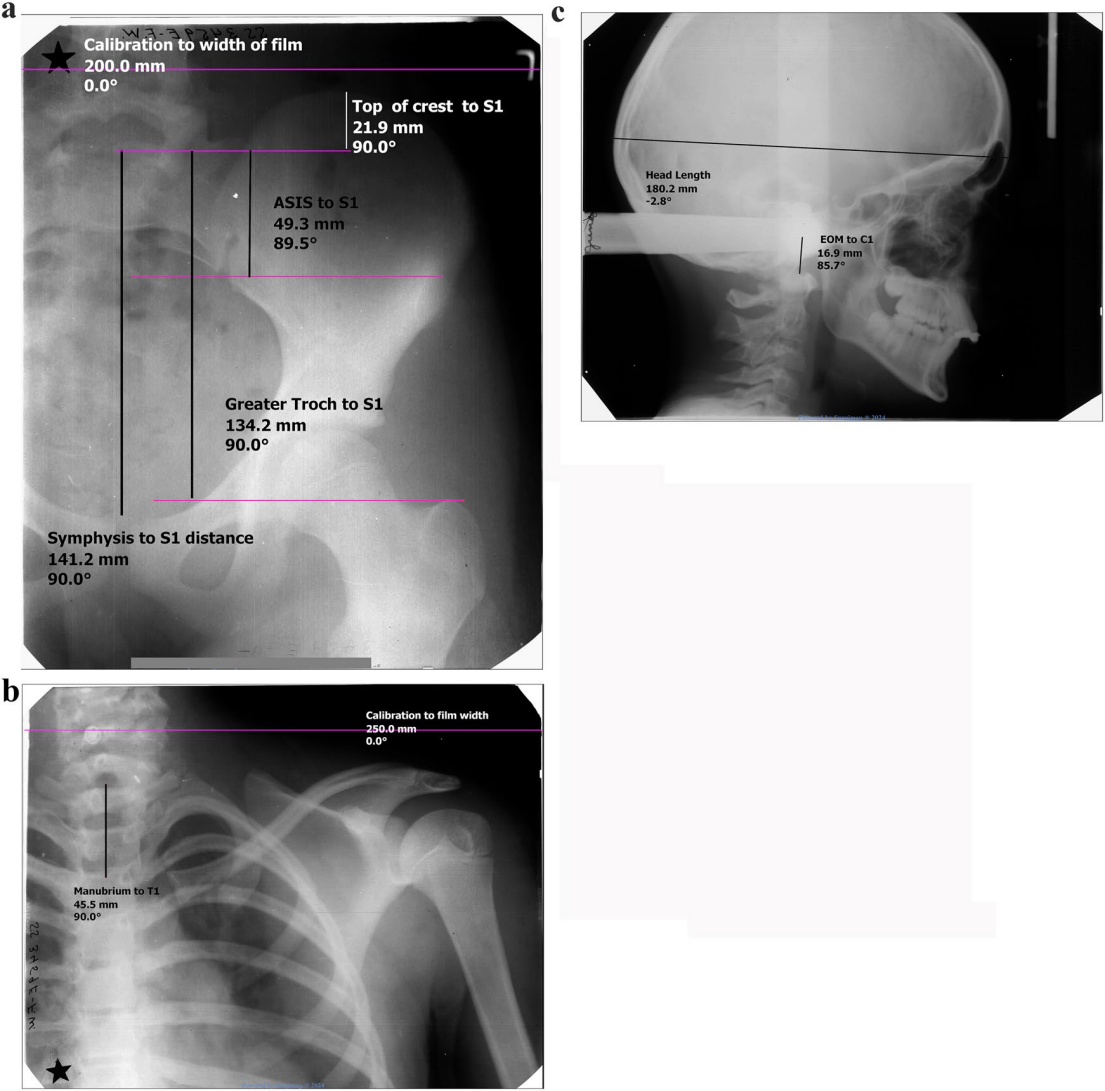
The radiographic measurements evaluating the distances from the pubic symphysis, iliac crest and ASIS to S1 from the left hip (A), the distance from the manubrium to T1 on the left shoulder (B), and the external auditory meatus to C1on the lateral skull radiographs (C)
